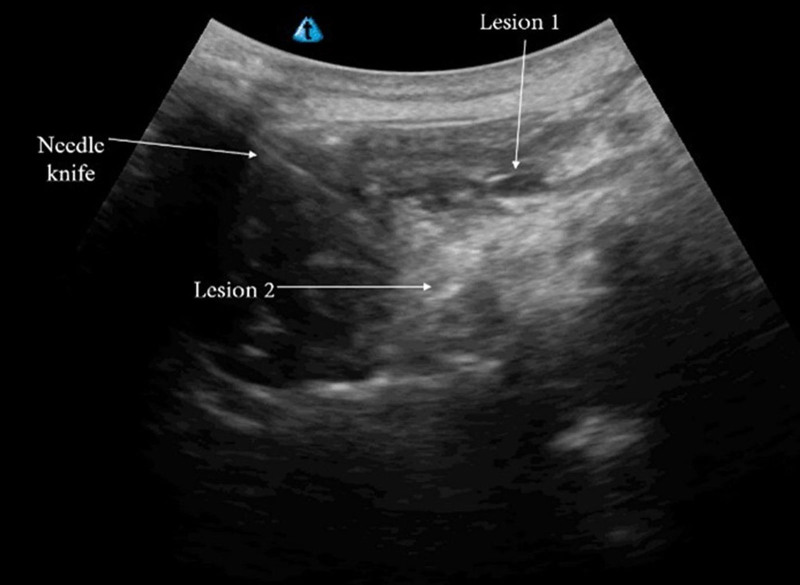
Multifidus muscle visualization under MU. MU = musculoskeletal ultrasound.
